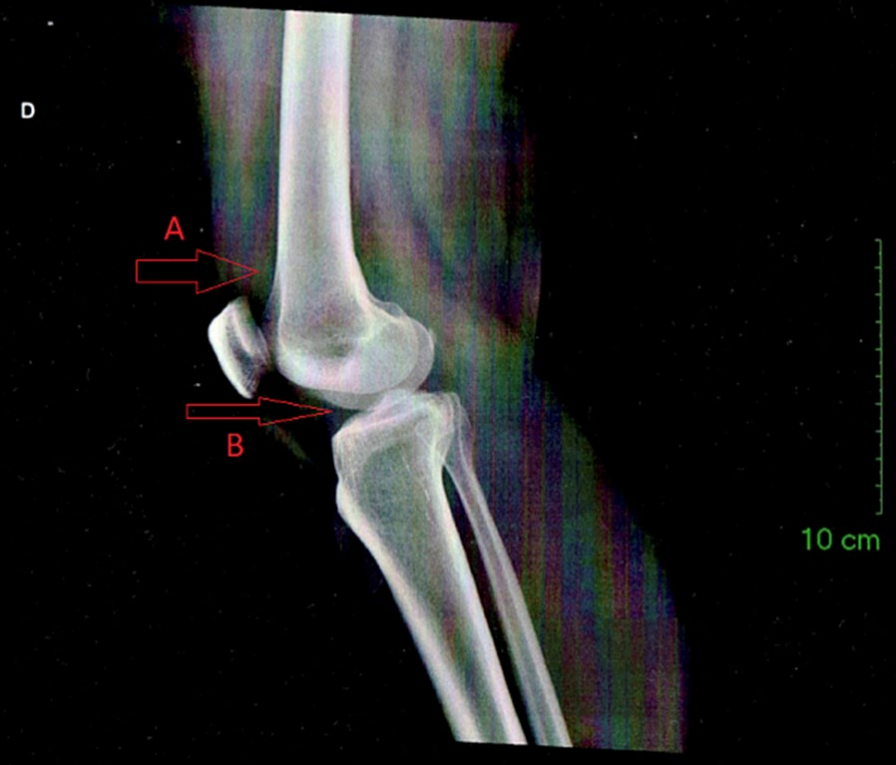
Right knee radiograph: suprapatellar synovial bursa opacification. The right knee radiograph displayed opacification of the suprapatellar synovial bursa, which is indicated by the *arrow A in the figure*, along with the narrowing of the medial compartment tibiofemoral joint in the right knee, indicated by the *arrow B in the figure*, and clinical picture, without any other alteration, indicates joint effusion, synovitis and active arthritis
